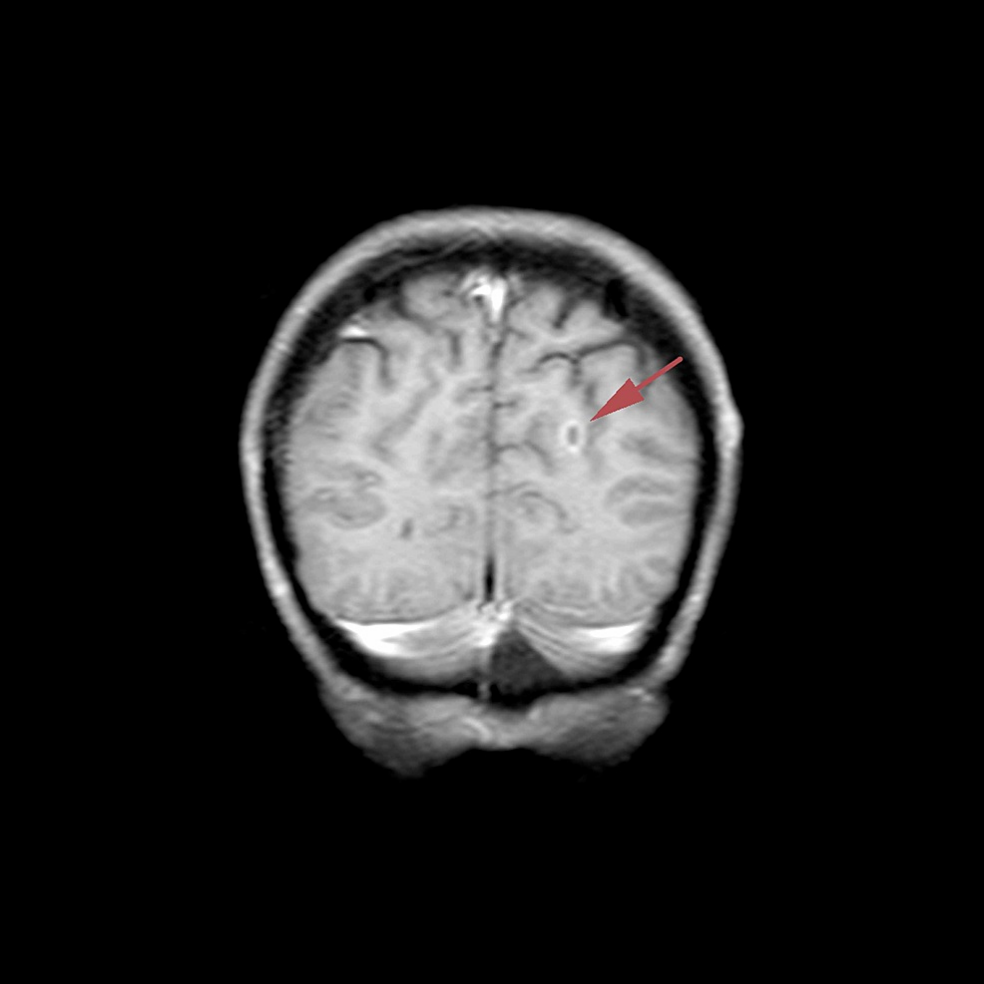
T1-weighted coronal brain magnetic resonance image with contrast dated November 5, 2018
